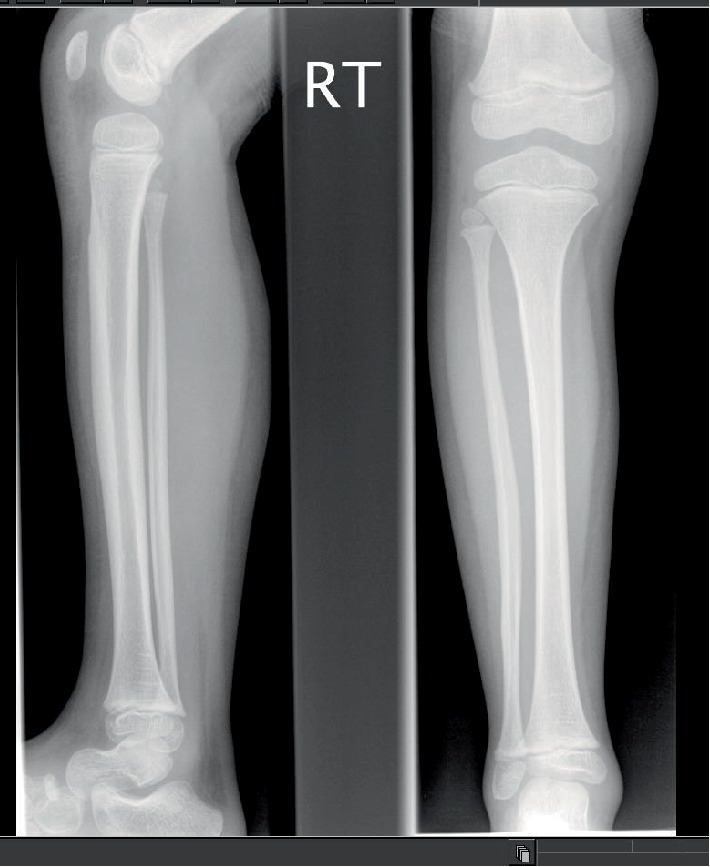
B1 radiograph of right lower limb (lateral and AP) aged 5 years.
